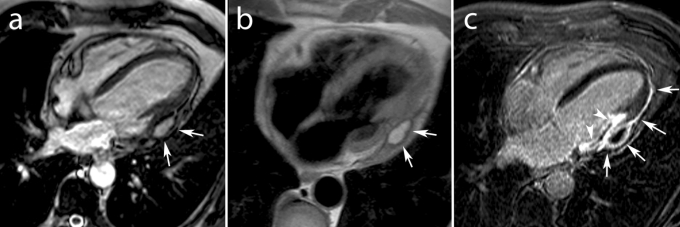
MRI study in a 66 year asymptomatic male with an old history of severe thoracic trauma (car accident) in which routine echocardiography showed an abnormal structure over the LV lateral wall. Horizontal long–axis cine (a) and T1–weighted (b) MRI show a well delineated, homogeneous and hyperintense pericardial mass (*arrows*, a,b). Late ce–MRI in the same imaging plane shows pericardial enhancement with the hypoenhancement of the mass (*arrows*, c) and adjacent transmural lateral myocardial wall enhancement (*arrowheads*, c). The combination of hyperintense signal on T1w with hypoenhancement on late ce–MRI is typical for old blood supporting the diagnosis of posttraumatic pericardial hematoma with secondary extrinsic compression and scarring on the adjacent LV wall.
